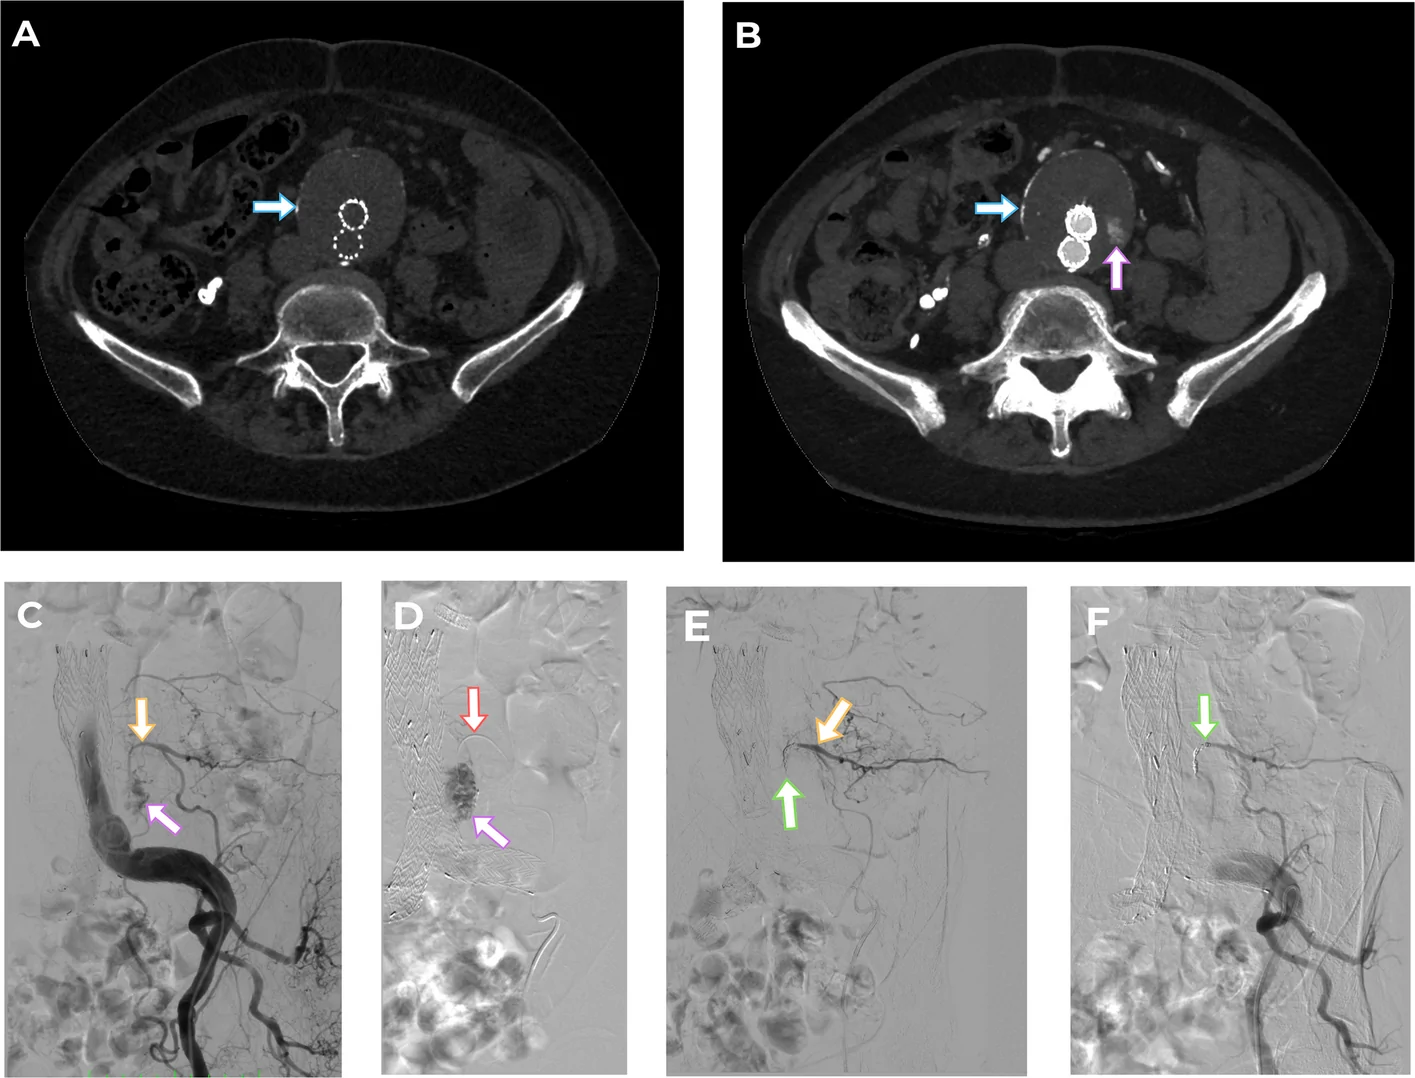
Imaging findings and procedural steps of transarterial coil embolization of a type II endoleak feeding vessel. A Unenhanced axial CT shows the aneurysm sac surrounding the endograft (blue arrow). B Contrast-enhanced CTA demonstrates the type II endoleak cavity (pink arrow) within the aneurysm sac (blue arrow). C DSA performed through a pigtail catheter demonstrates the feeding vessel (orange arrow) and opacification of the endoleak cavity (pink arrow). D Selective microcatheter DSA (red arrow) confirms filling of the endoleak cavity (pink arrow). E Selective angiography after coil (EV3 Concerto) deployment shows the coil pack within the treated feeding vessel (green arrow) and no residual opacification of the endoleak cavity; the feeding artery is indicated by the orange arrow. F Completion digital subtraction angiography confirms stable coil position (green arrow) and absence of residual endoleak filling. This example represents an anatomically simple endoleak with an accessible dominant feeder; immediate angiographic occlusion should not be considered equivalent to durable clinical success
